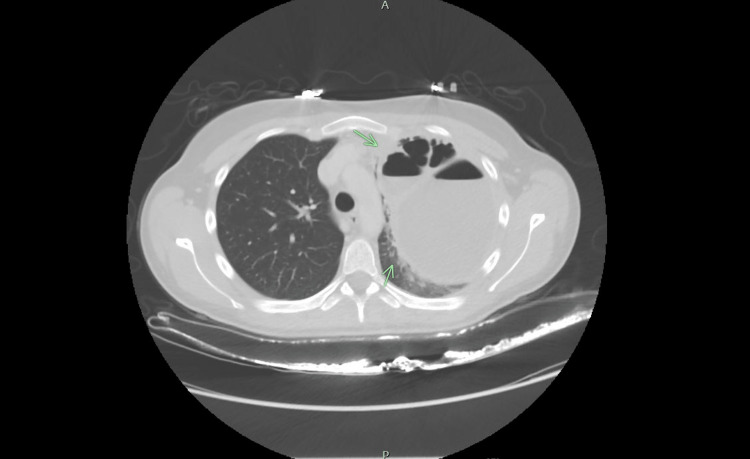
Chest CT on presentation showing a loculated left-sided lung abscess with air fluid levels
